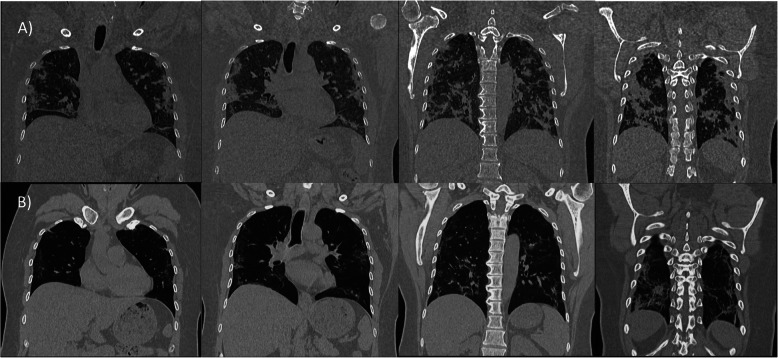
Case 51: 44-year-old female (healthcare worker), with a history of hypertension and hypothyroidism under treatment, score 4. CT chest: a day of admission (April 14th). Multi-focal areas of ground-glass opacification/consolidation in the bilateral lungs-lesions. b Day 5 after treatment (April 20th). The ground-glass opacities and organized consolidation is seen previously in both lungs in subpleural and peripheral locations appear to be less dense with less size comparing with the previous study done on 14 April 2020 suggestive of good responding to treatment with markedly resolving disease
